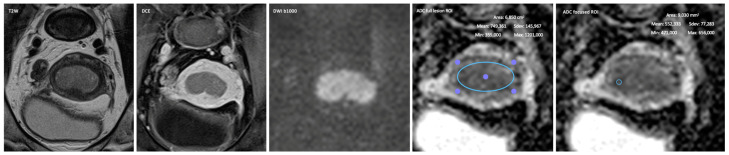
Sixty-year-old female staged at MRI as T1a and proven at pathology as T1a. Depiction of the method used for the quantitative data collection with the two different ROI—full lesion ROI (large circle) and focused ROI (small circle).
